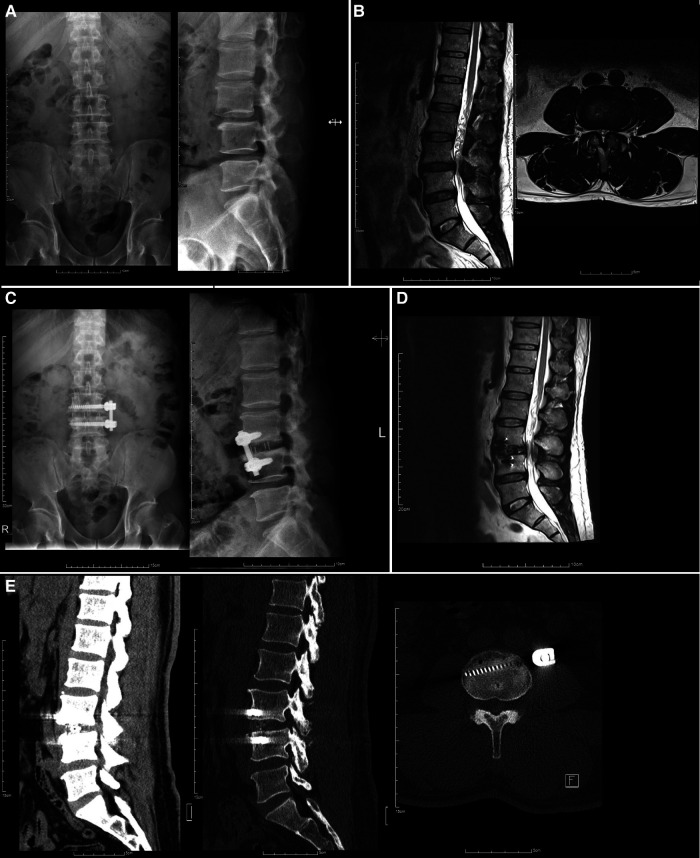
Preoperative and postoperative images of a 52-year-old man with L3-4 spondylolisthesis grade I who underwent one-stage OLIF. (A) Preoperative radiographs. (B) Preoperative MRI, (C) one-month postoperative radiographs, (D) 2-week postoperative MRI and (E) 5-month follow-up CT scan.
